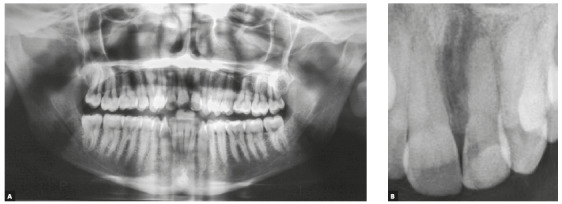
A) Initial panoramic radiograph. B) Initial periapical radiograph (tooth #22) shows complete pulp calcification.
